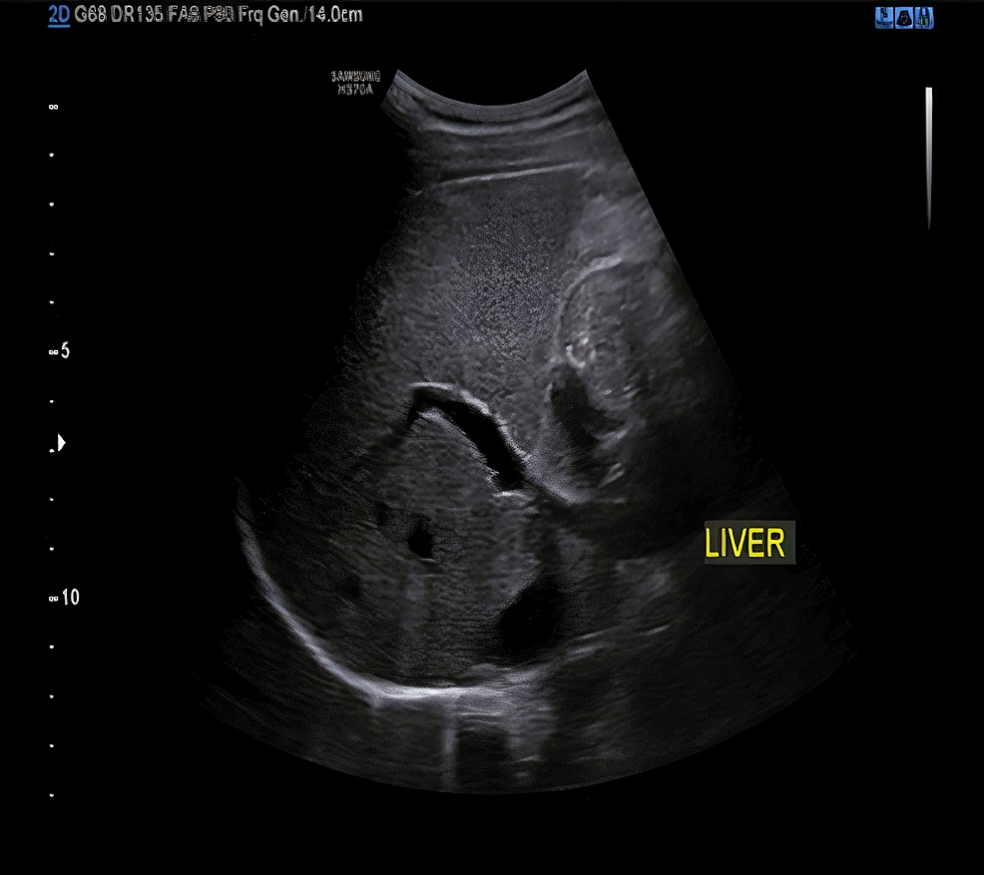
Ultrasound abdomen showing normal liver size and echotexture.
